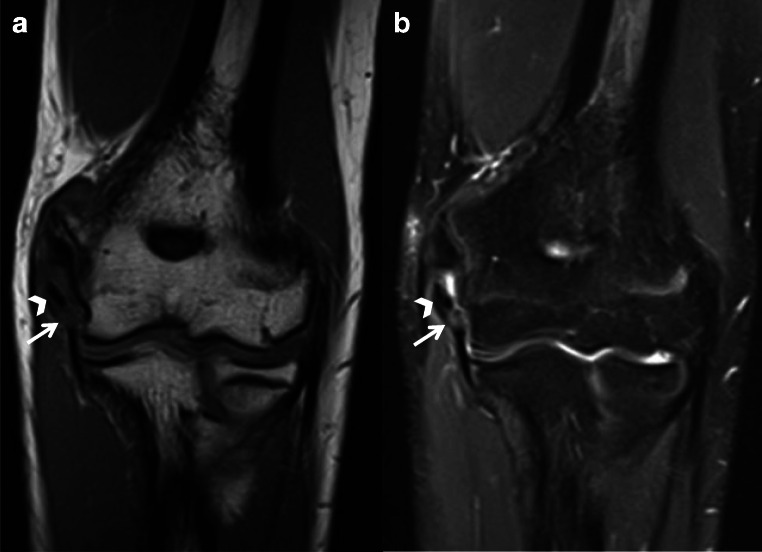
Axial T1 (a) and T2 (b) MR images of the elbow demonstrate an avulsed medial epicondyle apophysis (arrowheads) with distal distraction. There is also a low-grade partial thickness tear of the proximal UCL (solid arrows)
